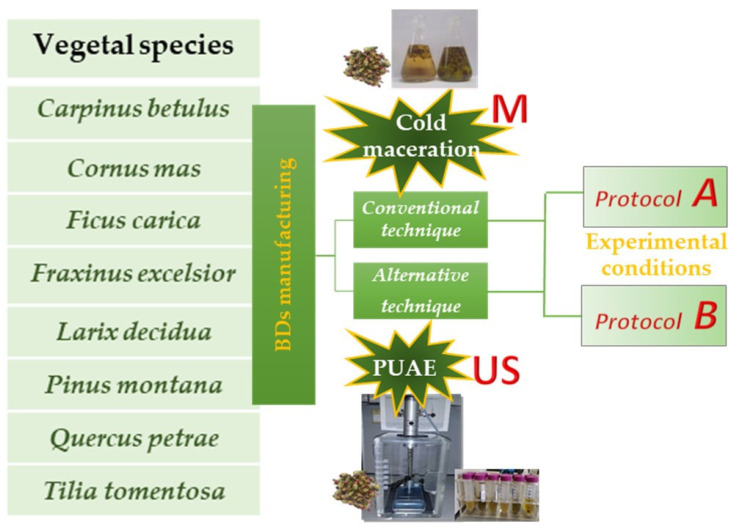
The global scheme of the experimental manufacturing of BDs: two methods (cold maceration, namely M, and ultrasounds, namely US) have been used. Each method has been applied following two different protocols (A and B).
